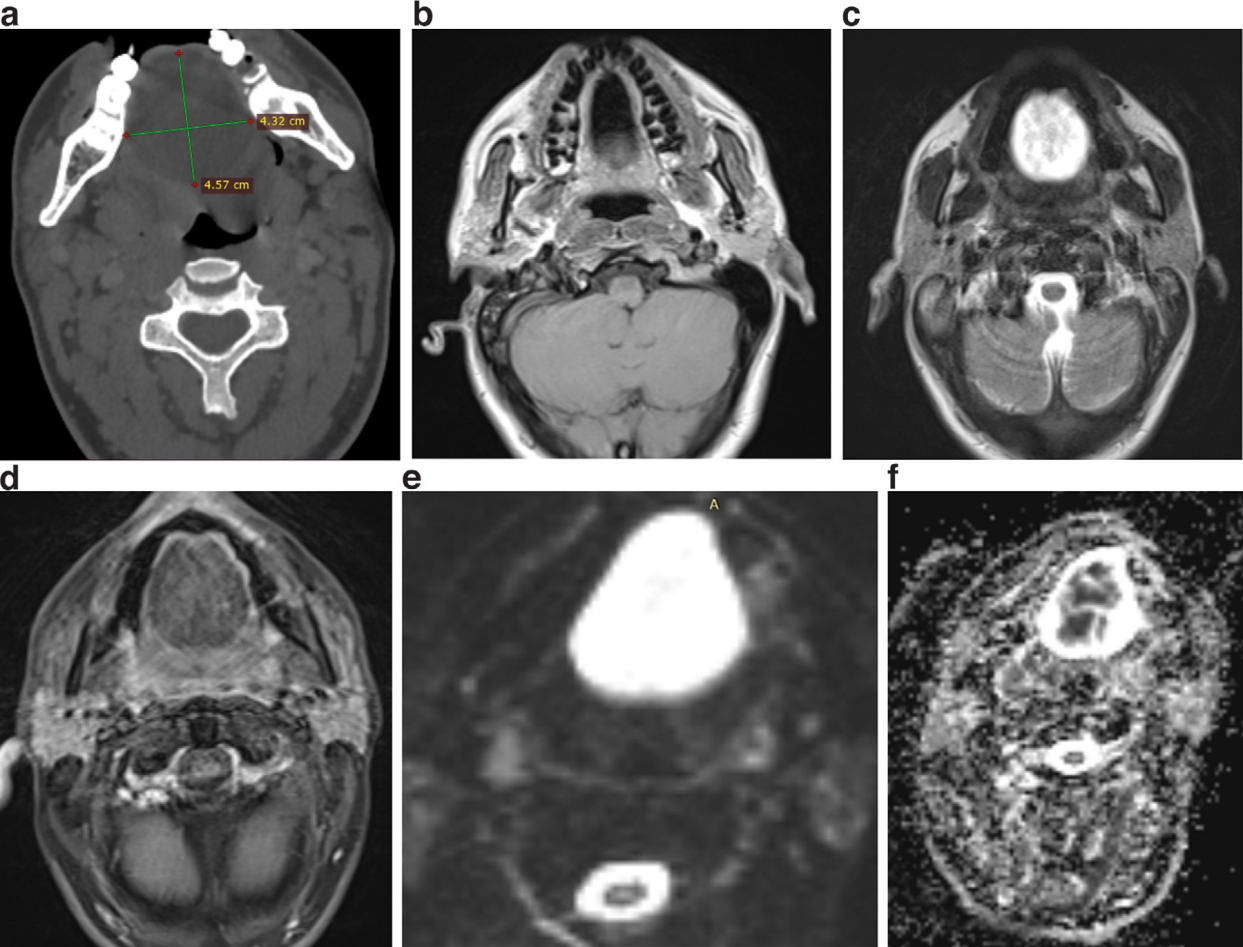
Imaging of 19-year-old female patient with sublingual dermoid cyst. Well-circumscribed in CT; (A) hypodense and well-circumscribed in MRI; (B) hypointense in T1WI; (C) hyperintense in T2WI, (D) no enhancement in STIR+C; (E) diffusion restricted in DWI; (F) extremely low SI in ADC; cystic lesion measured 41 × 45 × 65 mm.
